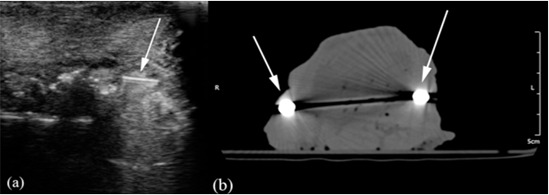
a: Arrow points to a metal foreign body on the ultrasound image. b: Arrows indicate two metal foreign bodies on the CT image (The dark streak in the middle of the image is caused by the beam hardening artifacts caused by the presence of bilateral metal particles).
